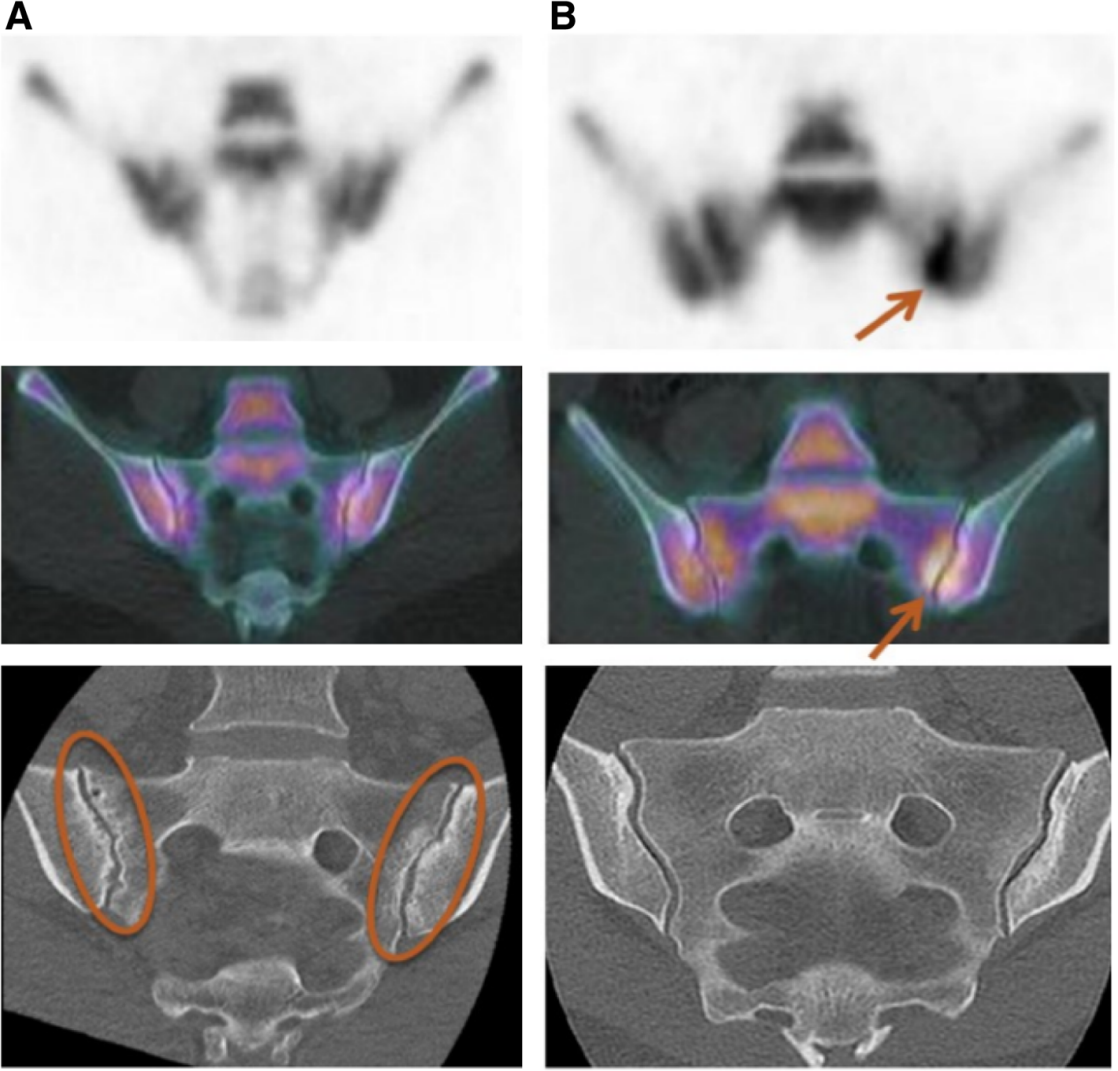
Two examples of discordance between PET/CT and CT scan. A negative PET/CT with structural sacroiliitis on CT scan and multiple erosions (a), and a positive PET/CT without structural lesions (erosion or ankylosis) on CT scan (b)
